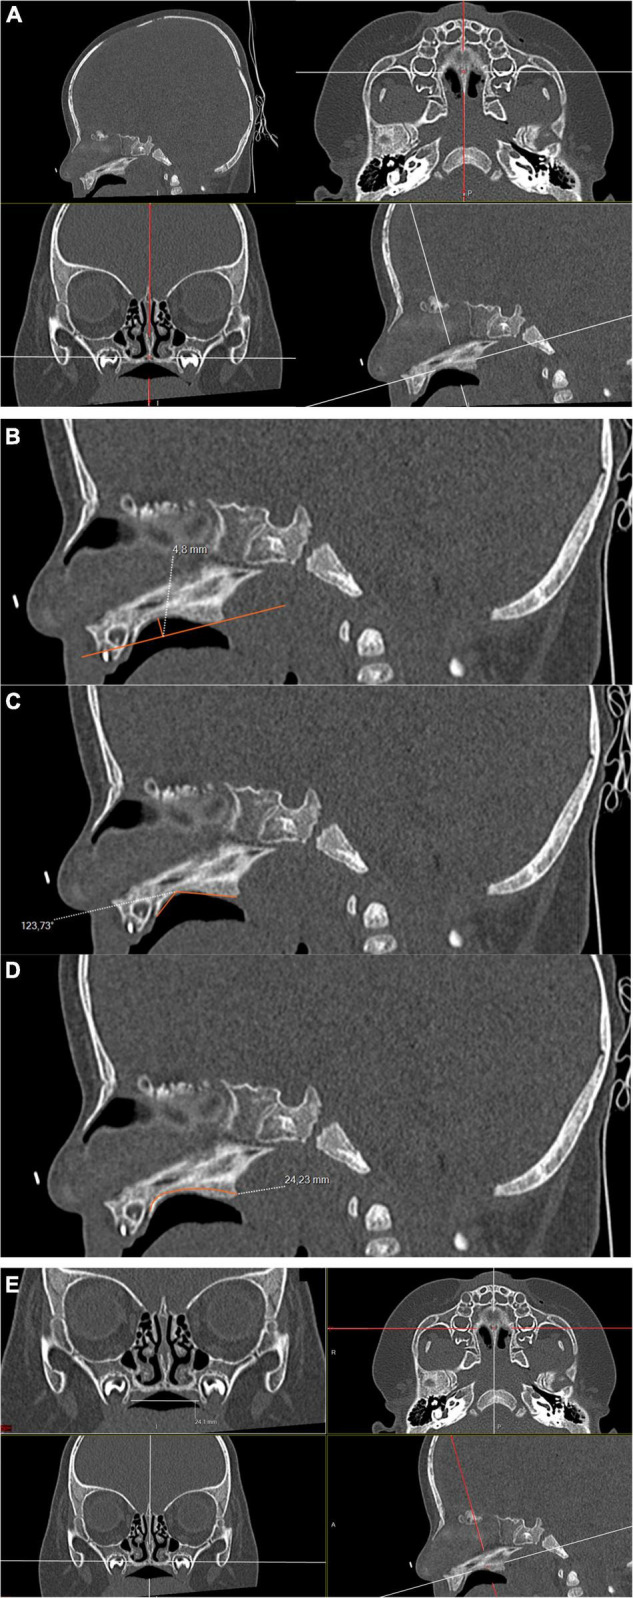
Head CT image of a 5-month-old deceased male with no specific cause of death found after post-mortem investigations; bone filtered, double oblique multiplanar reconstruction; 100 kV, 95 mAs. (A) Sagittal plane used to measure the height (B), the sagittal angle (C), and the length of the hard palate (D). (E) Coronal plane for the measurement of the width of the palate.
